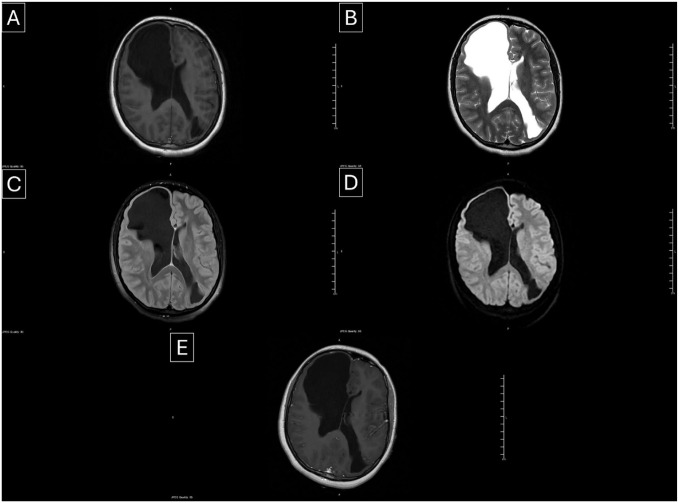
(a–e) Axial MRI of the brain demonstrates bilateral porencephalic cysts: (a, T1-weighted) CSF-intense cystic lesion in the right frontal lobe communicating with the frontal horn of the right lateral ventricle, with associated cortical thinning. A similar CSF-intense cyst is seen in the left occipital lobe communicating with the occipital and temporal horns of the left lateral ventricle, (b, T2-weighted) the cysts appear hyperintense, clearly delineating their extent and ventricular communication, (c, FLAIR) perilesional hyperintensity consistent with gliosis is seen adjacent to the right frontal cyst, while no surrounding gliosis is noted around the left occipital cyst, (d, diffusion-weighted imaging) no diffusion restriction is identified within either lesion, and (e, post-contrast T1-weighted) no abnormal enhancement is observed. Mild mass effect with subtle leftward midline shift is noted. R/L orientation markers are visible in all panels. CSF: cerebrospinal fluid; MRI: magnetic resonance imaging.
